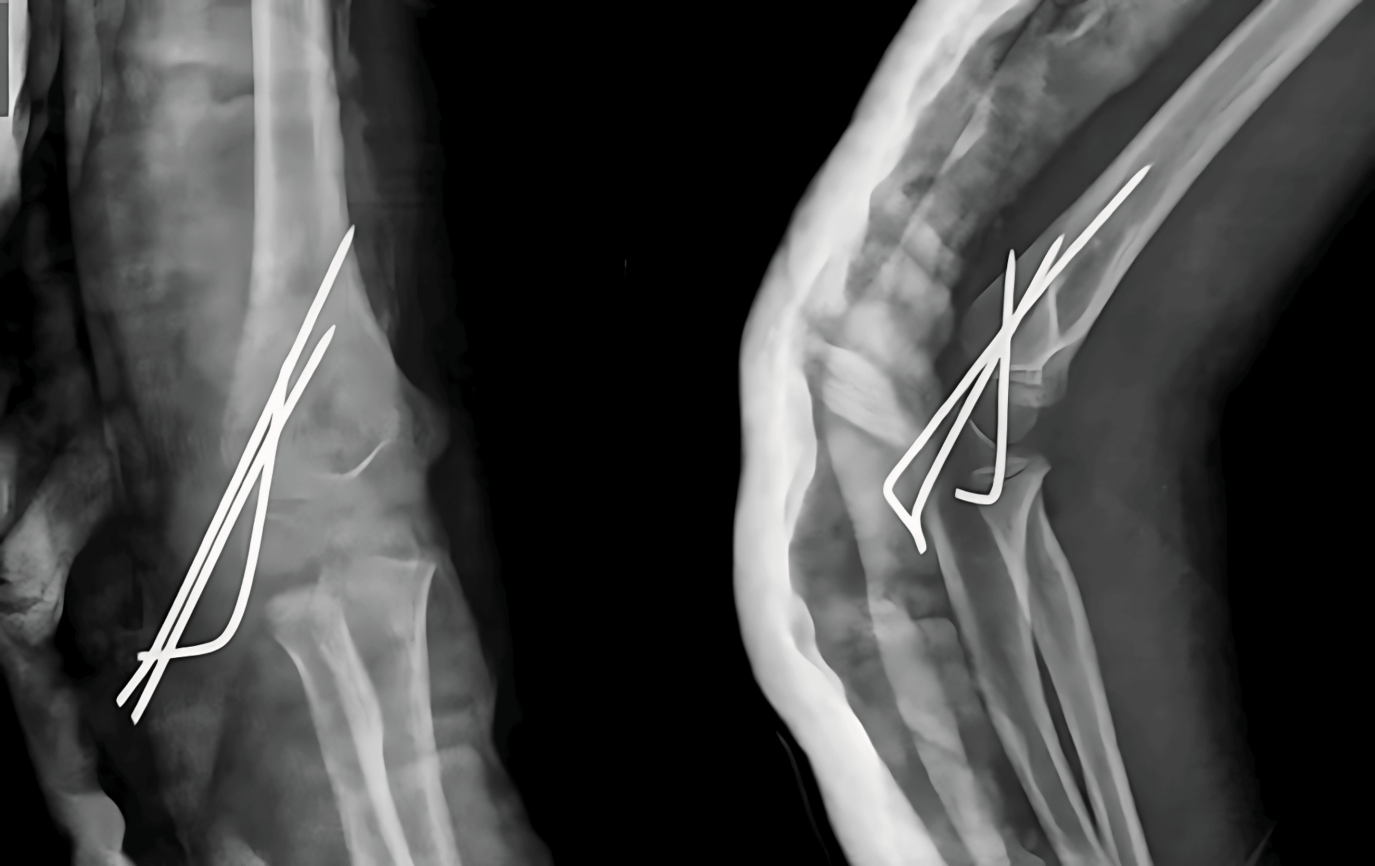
Postoperative X-ray of case 2
